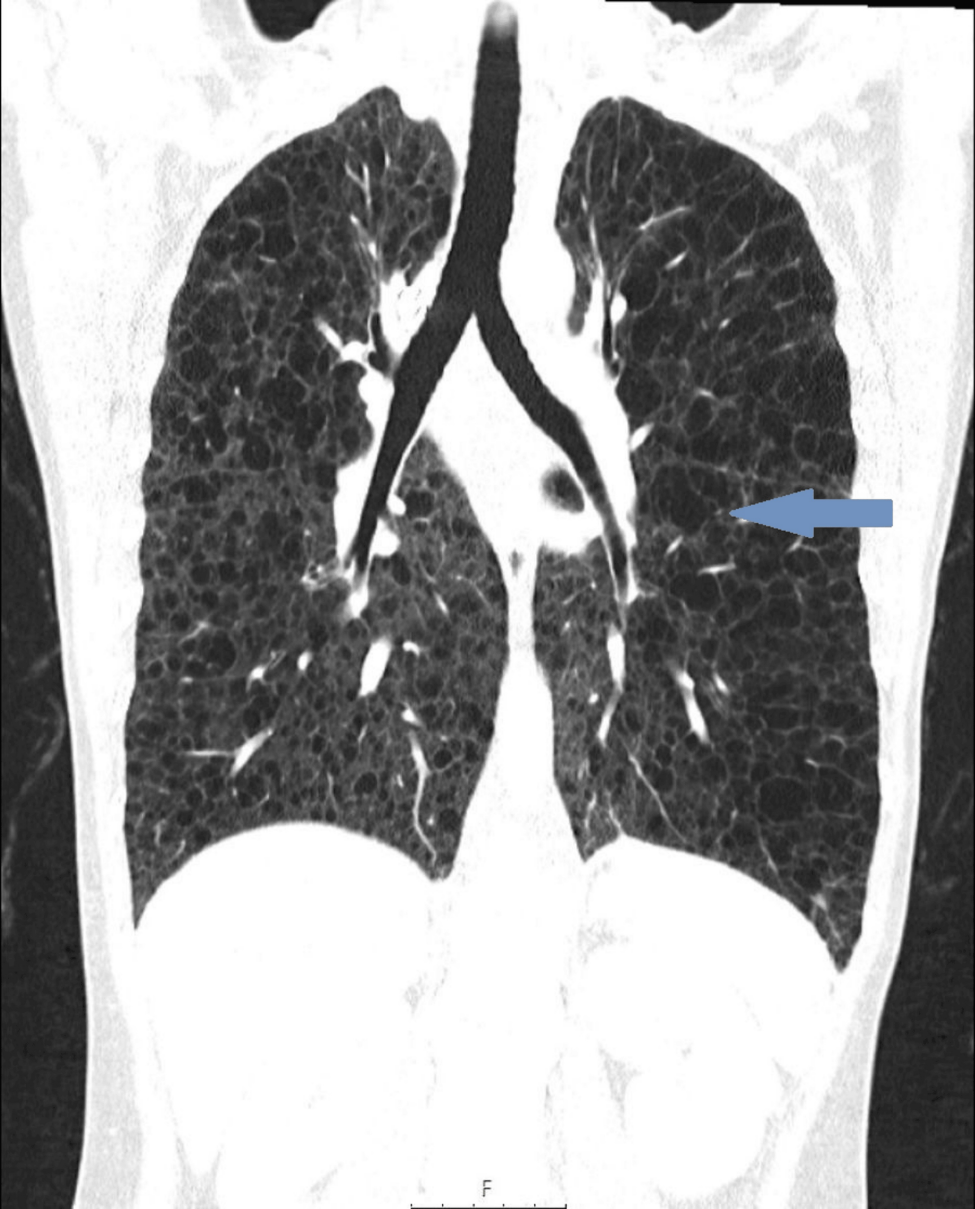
Thoracic computed tomography scan showing diffuse intrapulmonary cysts in coronal view (arrow).
